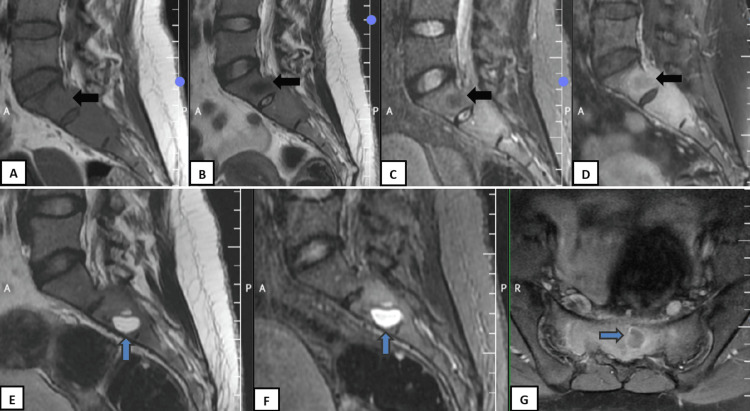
A panel of MRI images showing sagittal (A) T1WI, (B) T2WI, (C) STIR, (D) T1 post contrast, (E) T2WI, (F) STIR, (G) Axial T1 post contrast. A small ill-defined area (black arrow) is noted within S1 appearing T1 isointense and T2/STIR hypointense, demonstrating mild post-contrast enhancement, suggestive of solid tumor components. The cystic component (blue arrow) is hyperintense on T2-weighted imaging (T2WI) and short-tau inversion recovery (STIR) sequences and peripherally enhancing on the post contrast study.
